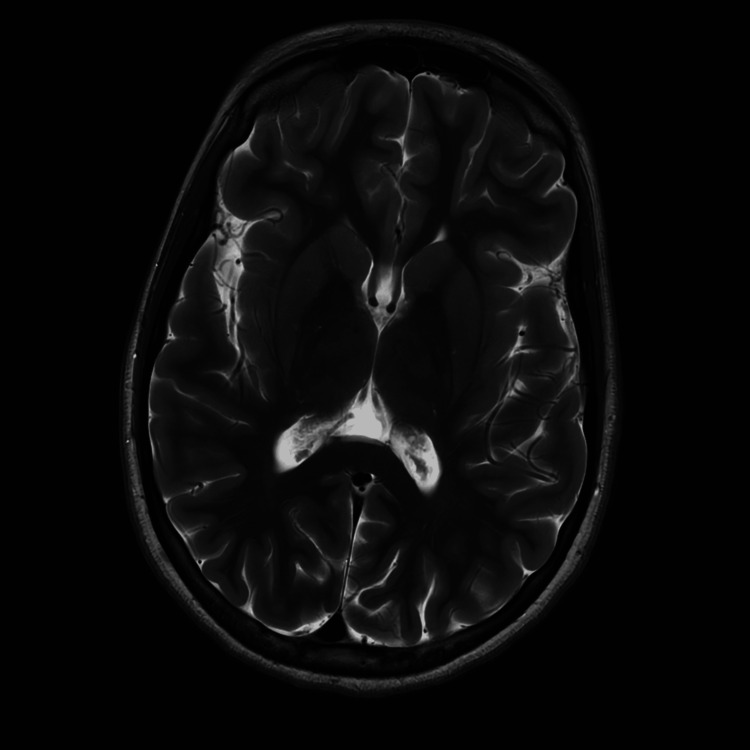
MRI of the brain with contrast showing no acute intracranial abnormality or pathologic enhancement
